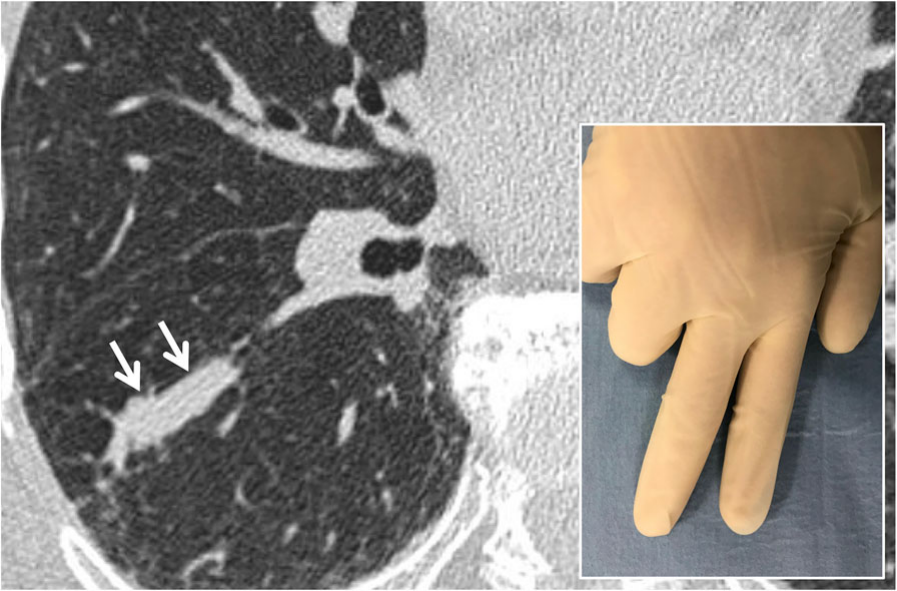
Finger-in-glove sign. Axial CT scan of a patient with bronchocele—located in the right lower lobe (white arrows). A bronchocele consists of a pulmonary opacity with characteristic shape (linear, V or Y shape)—with well-defined lobulated margins: the appearance is similar to that of a finger-in-glove
